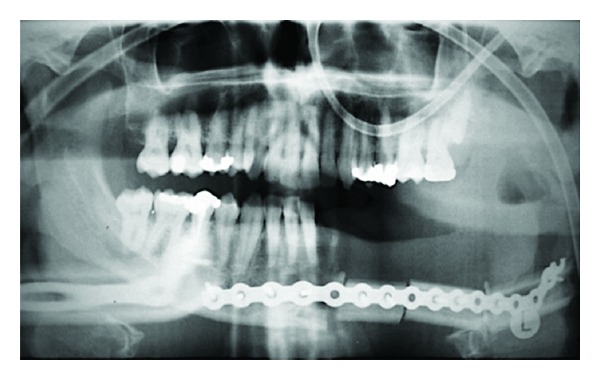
Immediately postsurgical treatment panoramic X-ray, which shows fibula free flap reconstruction of the mandible.
